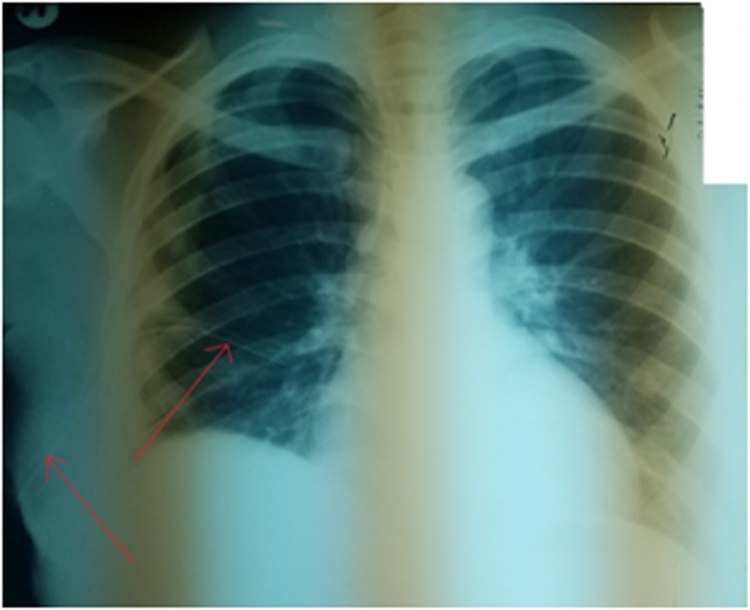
Chest X-ray showing resolving right-sided pleural effusion post Thoracostomy. Arrows show chest tube in situ.
